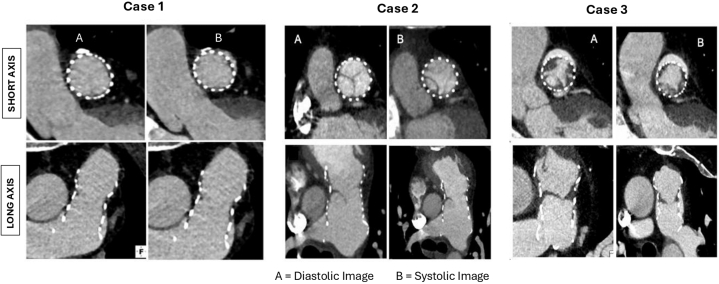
2-Dimensional Cardiac Computed Tomography Images Two-dimensional images in short-axis and long-axis in diastole (A) and systole (B). Case 1 shows an example without hypoattenuating leaflet thickening (HALT). Case 2 shows mild HALT on a single valve leaflet motion. Case 3 shows significant HALT on all 3 leaflets with concomitant restricted leaflets motion.
